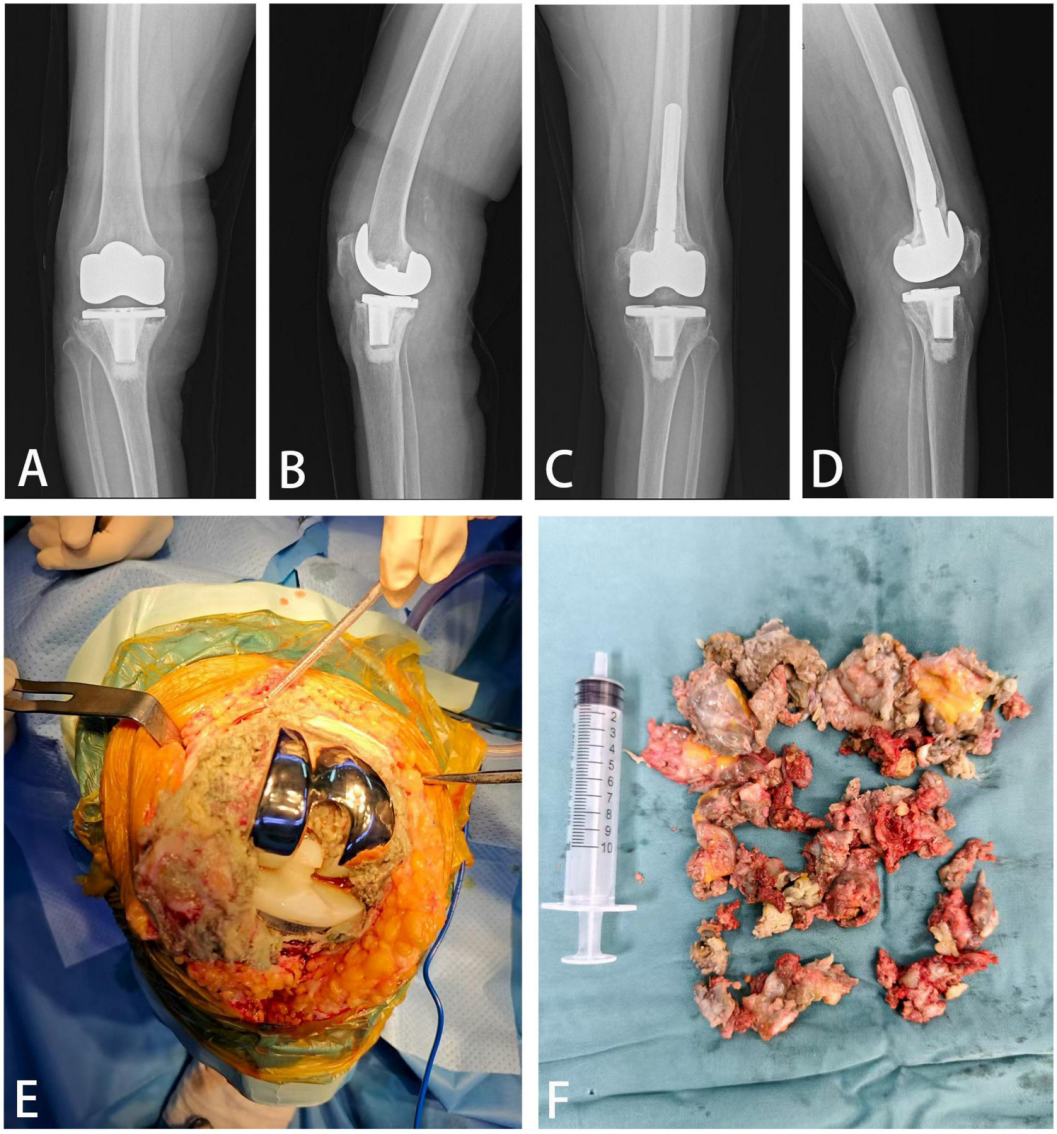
(A) The 2023 anteroposterior x-ray after initial total knee arthroplasty. (B) The 2023 lateral x-ray of the right knee. (C) The 2023 follow-up anteroposterior x-ray after left knee revision surgery. (D) The 2023 lateral follow-up x-ray of the left knee. (E) Intraoperative view of synovial hyperplasia. (F) Resected nodular synovium.
